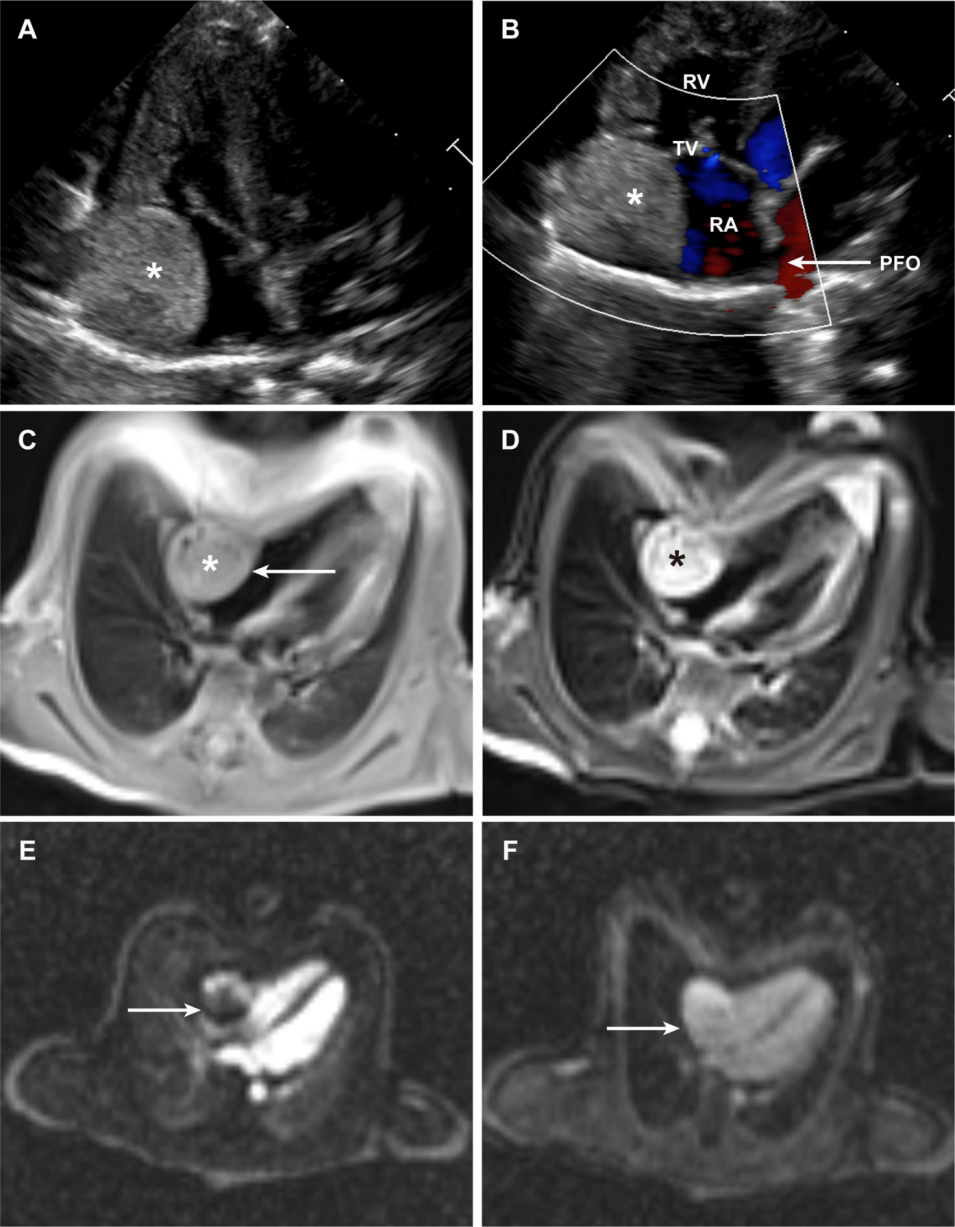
Clinical images of the patient. (A) Transthoracic echocardiographic image of the intracardiac hemangioma. (B) Color Doppler image demonstrating intracardiac blood flow. (C,D), Cardiac MRI image of the intracardiac hemangioma in the T1-weighted (C) & T2-weighted (D) sequence. (E) Cardiac MRI image of the intracardiac hemangioma from first-pass perfusion imaging. (F) Cardiac MRI image of the intracardiac hemangioma from delayed enhancement imaging. (PFO, patent foramen ovale; RA, right atrium; RV, right ventricle; TV, tricuspid valve).
